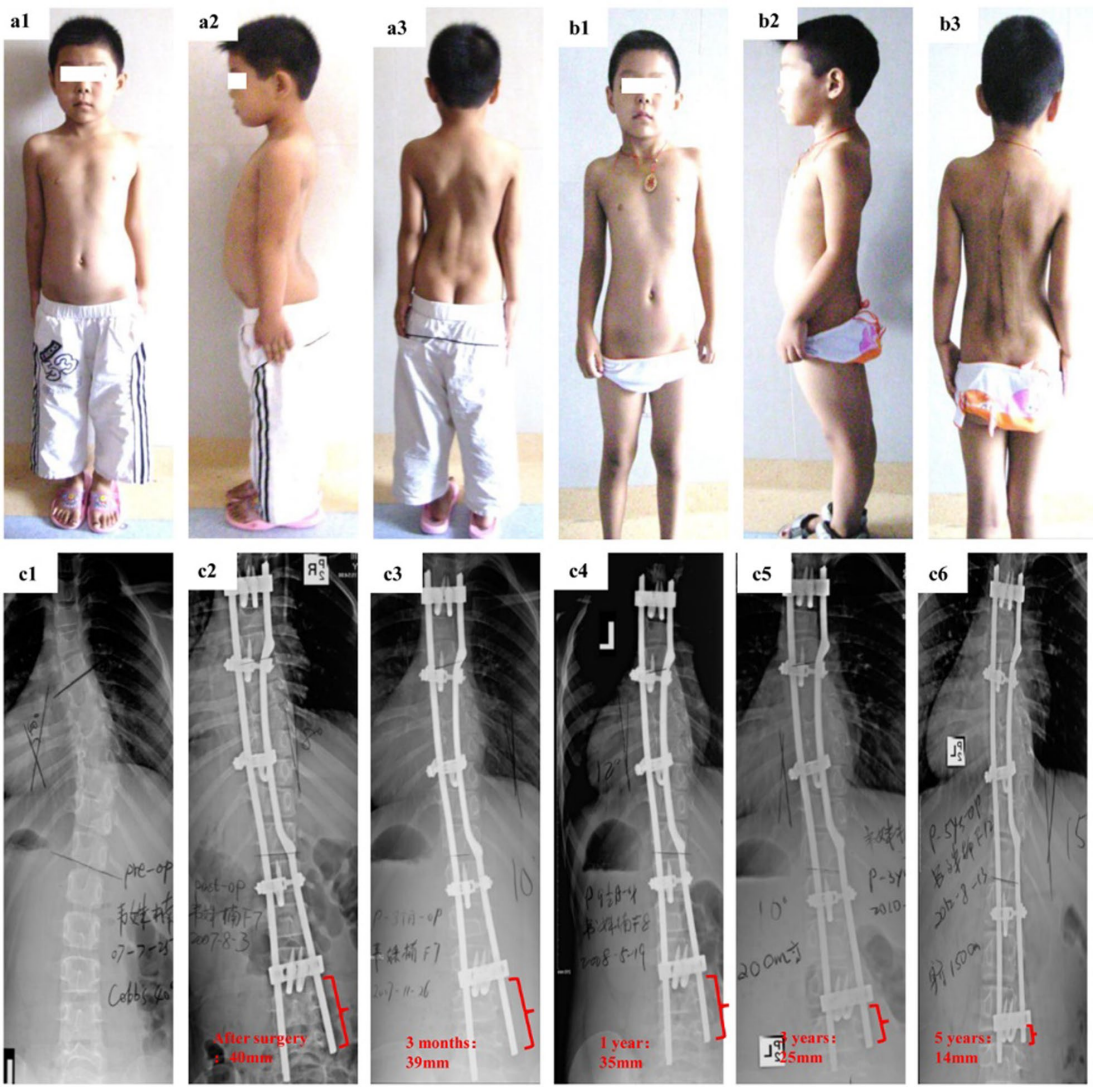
A 5-year-old boy with progressive early onset scoliosis treated by the plate-rod spinal system. a1–a3 Preoperative photographs. b1–b3 Postoperative photographs. c1–c6 On plain radiographs, c1 the preoperative Cobb angle is 40°. c2 The postoperative Cobb angle is 8° and the postoperative rod tail reserve length is 40 mm. c3–c6 The rod tail length at 3 months, 1 year, 3 years and 5 years postoperatively is 39 mm, 35 mm, 25 mm and 14 mm
